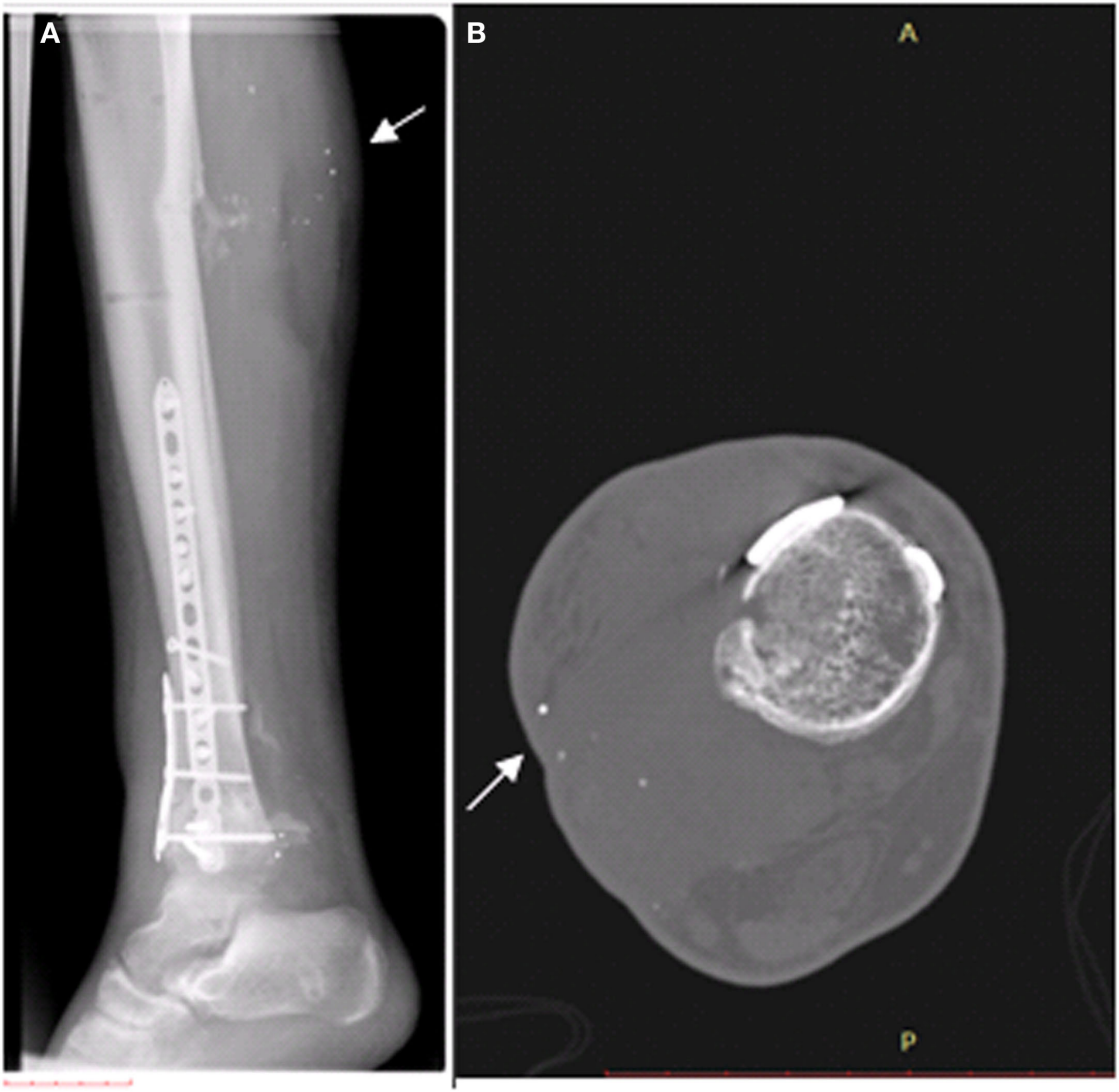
Existence of metal fragments (white arrows), which were not removed during the surgical procedures, in the right calf. (A) X-ray preformed 1 year after the injury. (B) CT scan preformed 3 years after the injury.
